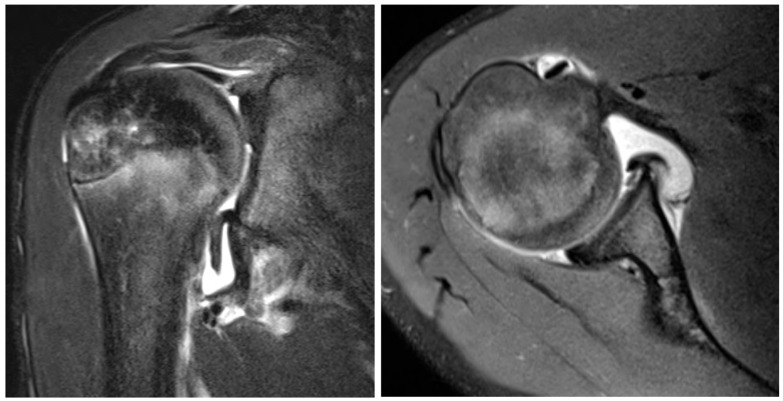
T2 weighted MRI coronal (left) and axial (right) slice of a 13-year-old boy with GLAD, SLAP, HAGL, and labral lesions.
